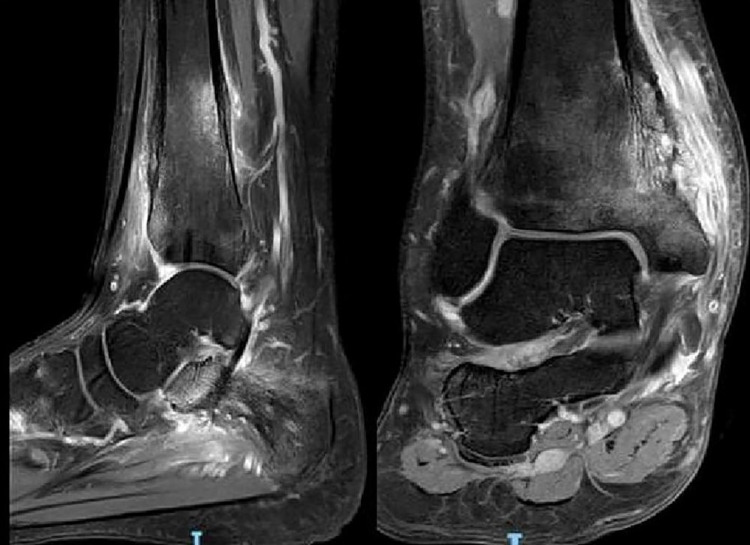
MRI of Left and right ankle. Erosions along the medial cortex of the distal tibial metaphysis and epiphysis with florid periosteal reaction and marked surrounding soft tissue edema. Bony edema also noted within the talus, calcaneum, navicular, medial cuneiform and base of first metatarsal. Findings are fairly symmetrical.
